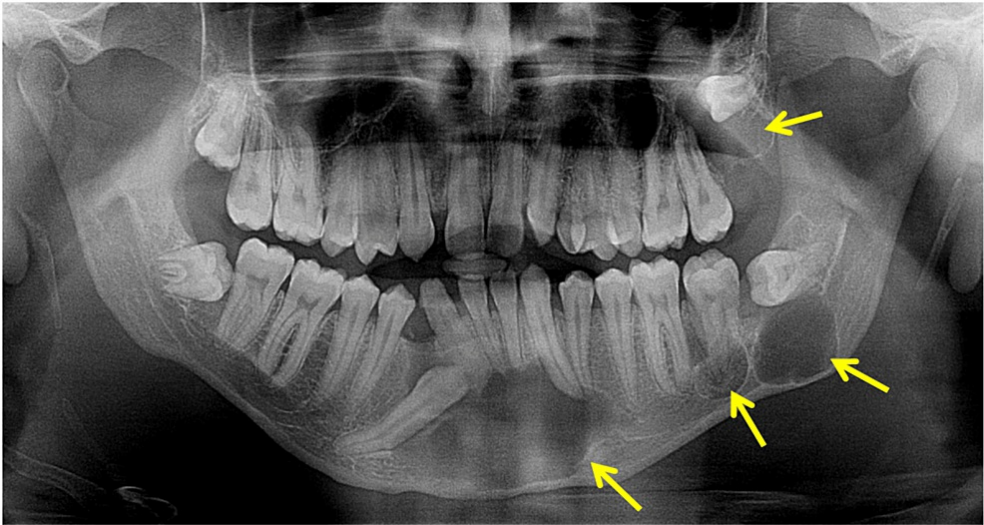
Orthopantomogram showing multiple cysts both in the mandible and one cyst in the maxilla
